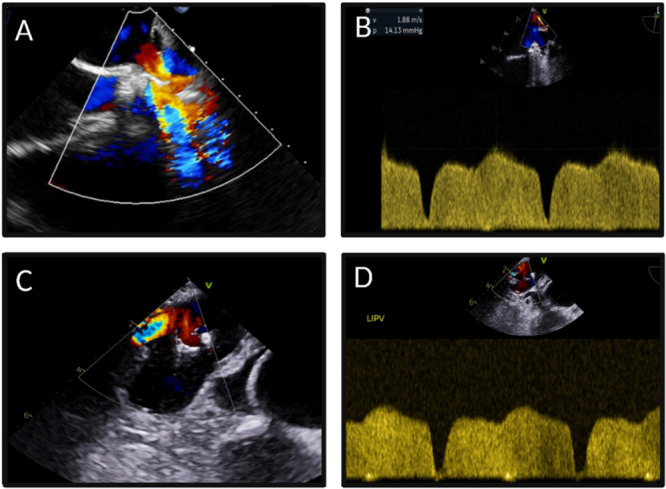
Transesophageal echocardiogram (TEE) in the diagnostic work‐up of patient 2. TEE revealed an important flow acceleration mainly in the LSPV with aliasing at color Doppler (A), and a peak velocity of 2 m/s, a peak gradient of 14 mmHg (B), suggesting pulmonary vein stenosis. Similarly, flows were accelerated in the LIPV (C), with a velocity of 1.6 m/s (D). [Color figure can be viewed at wileyonlinelibrary.com]
